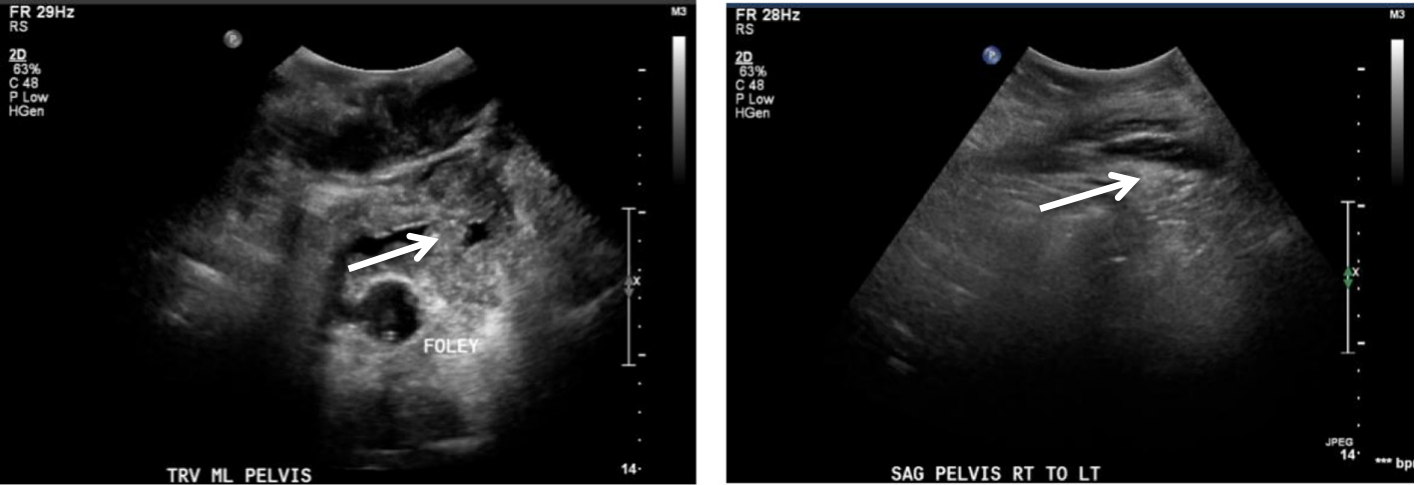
Ultrasonography of the upper pelvis, transverse (left) and sagittal (right) views. The heterogeneous mass (arrows) lies superficial to the bladder, which is decompressed with a Foley catheter.
